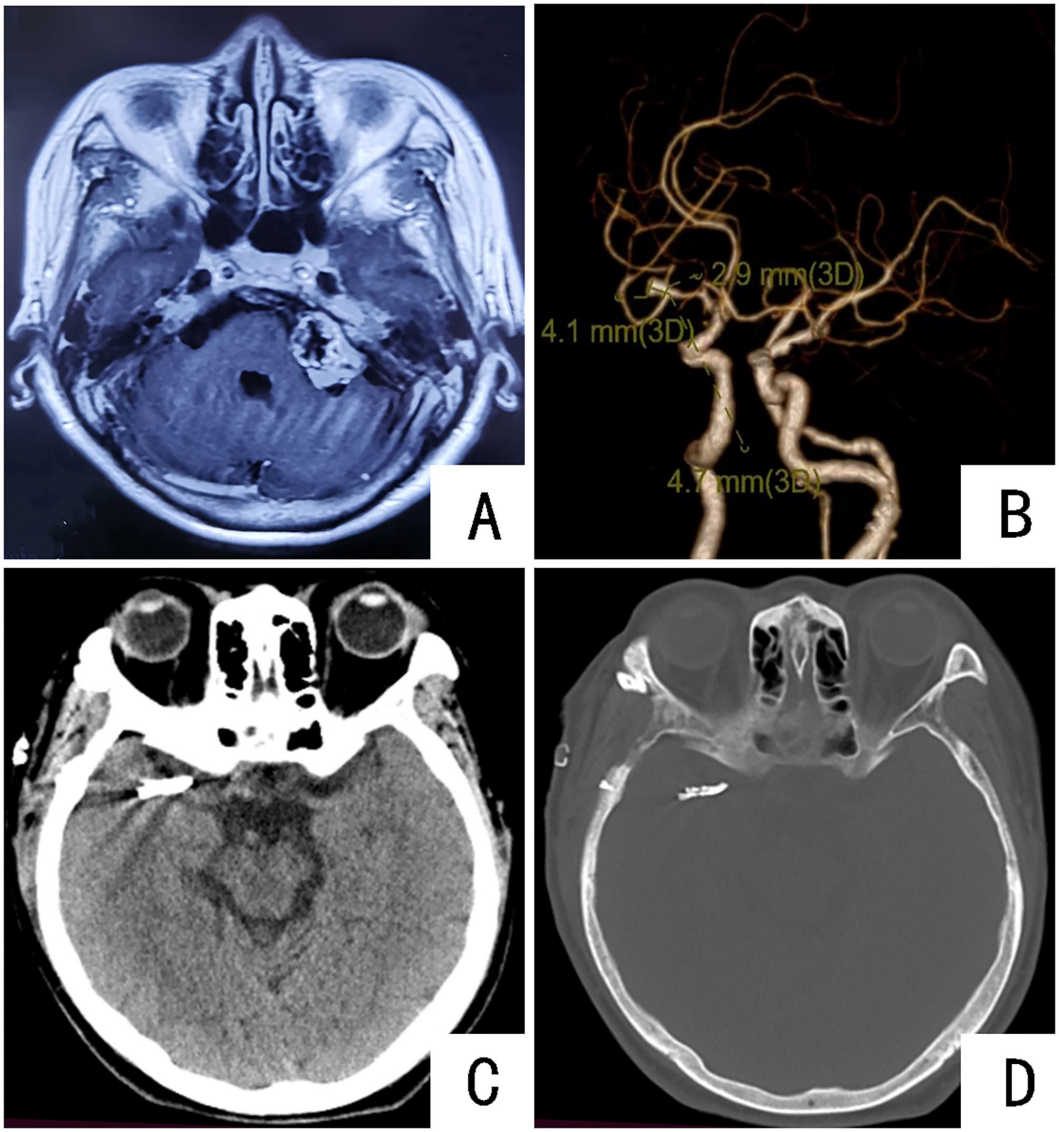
Preoperative and postoperative imaging examinations of the patient: (A) MRI showing space-occupying lesions in the left cerebellopontine area; (B) CTA showing the right middle cerebral artery M1 aneurysm; (C,D) CT results of the head of the patient on the first day after the operation. Aneurysm clippings can be seen.
